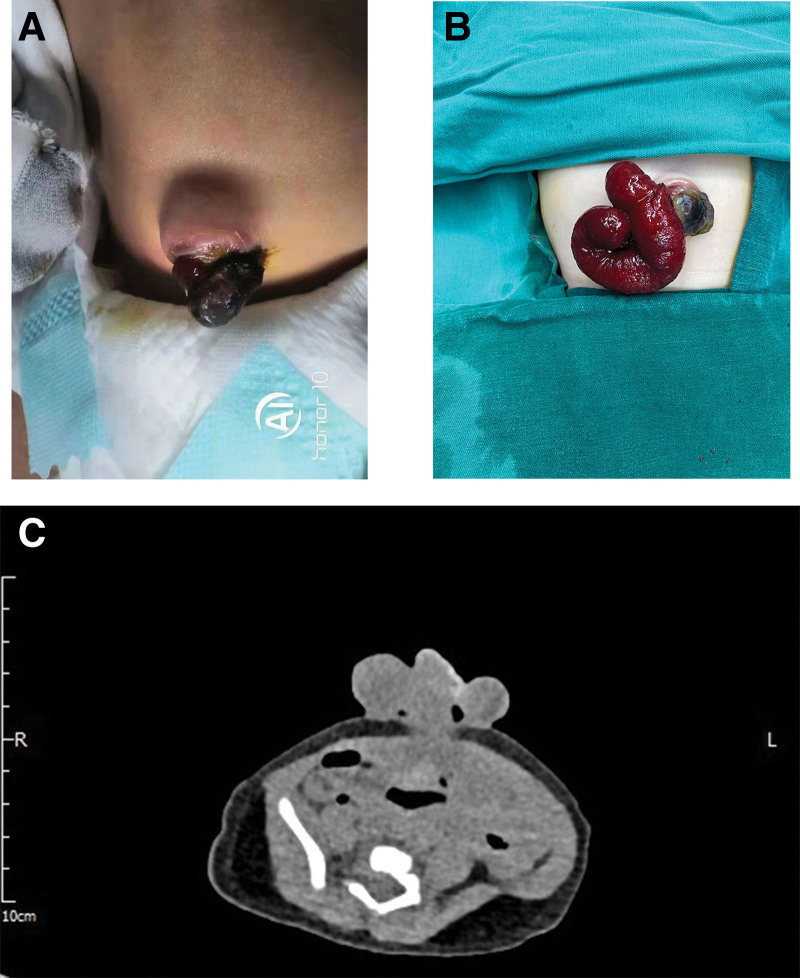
Umbilical lesions. (A) Red mass in the umbilical cord; (B) the red mass protruding from the umbilical cord gradually increased in the shape of “Y”; (C) CT imaging examination of the lesion.
